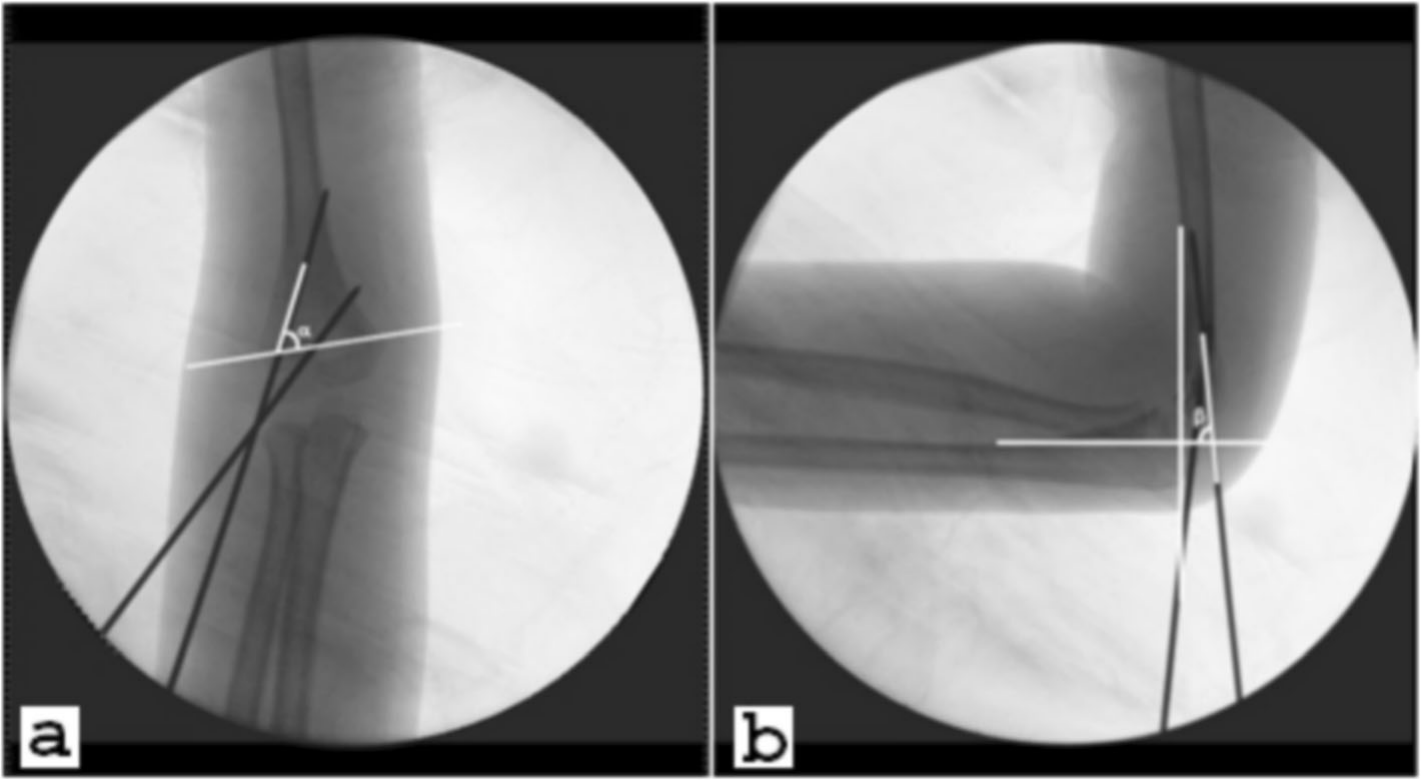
Pin angles measured on the intra-operative radiographs. a Coronal pin angle *α* is formed by the proximal pin and a line traversing the maximum diameter of the olecranon fossa (inter-epicondylar line). b Sagittal pin angle *β* is formed by the proximal pin and a line at the most distal ossified humeral bone edge, which is perpendicular to the anterior humeral line
